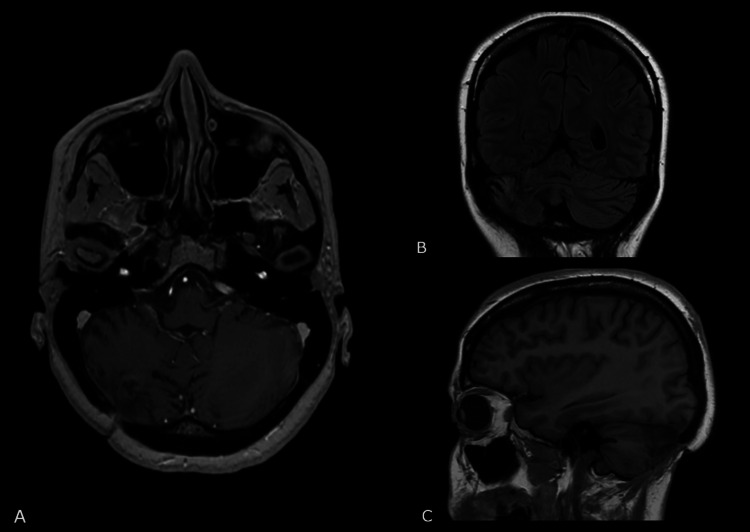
Follow-up magnetic resonance imaging two years after surgery (A: axial; B: coronal; C: sagittal).
